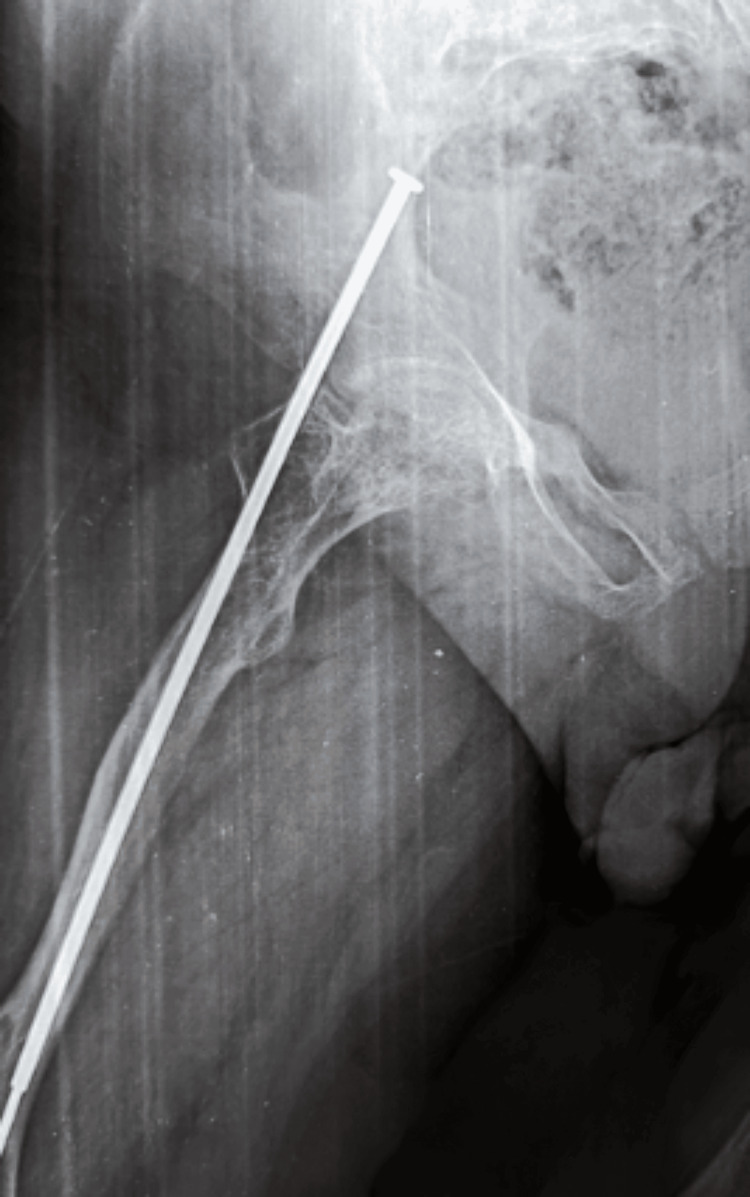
Radiograph showing telescopic nail migration into surrounding soft tissues This complication may occur due to poor initial anchorage or trauma, highlighting the need for meticulous technique.
